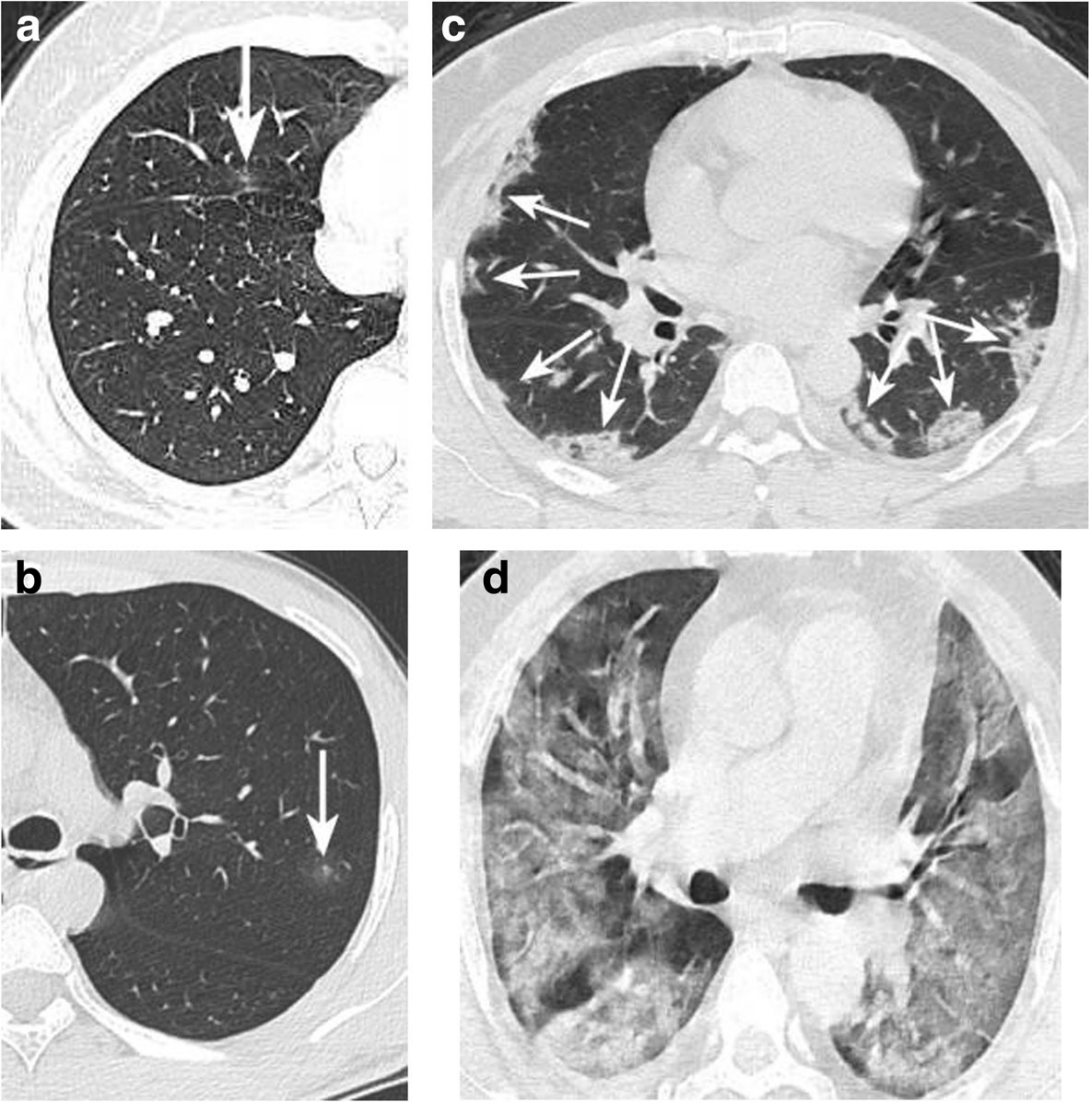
Atypical case a: Mild type patient. CT showed a small pGGO in the right middle lobe (arrow), and no abnormality in the remaining lobes. Atypical case b: Mild type patient. Only a small pGGO was detected in the left upper lobe with hale sign (arrow). Typical case c: Moderate type patient. CT showed multiple GGOs in the bilateral lobes with vascular dilatation and halo sign (arrow). Typical case d: Heavy type patient. CT showed diffused GGOs in the bilateral lobes
